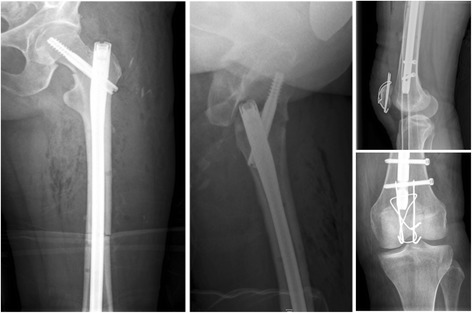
Follow-up radiographs of left hip at day 3 post-op, showing satisfactory reduction and fixation of the left femoral neck and shaft fractures by intramedullary nail and the patella fracture, respectively
